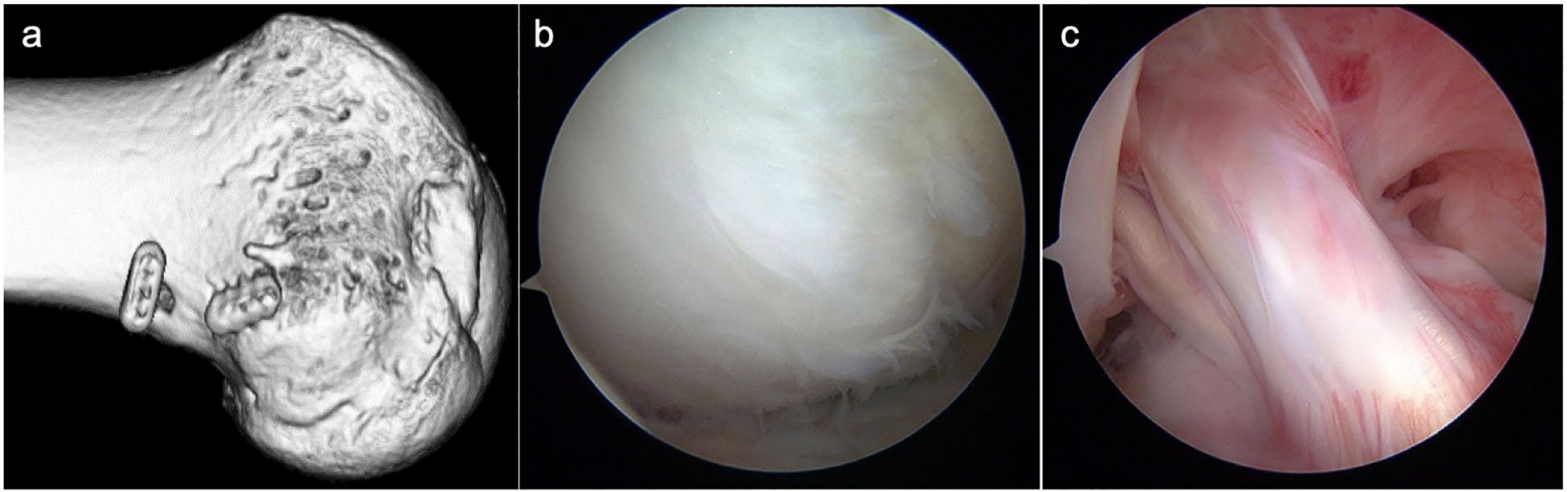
Postoperative imaging of computed tomography and second-look arthroscopic appearance. A 3-dimensional computed tomography of the lateral femoral condyle from a lateral side view at 13 months after surgery. a Postoperative image showed the contour of the lateral femoral condyle was reconstructed with the implanted iliac bone graft. b Smooth cartilaginous tissue with a slightly fibrillated surface was observed at the implantation site of the lateral femoral condyle. c Reconstructed anterior cruciate ligament grafts had no laceration or elongation and was wholly covered with synovium
